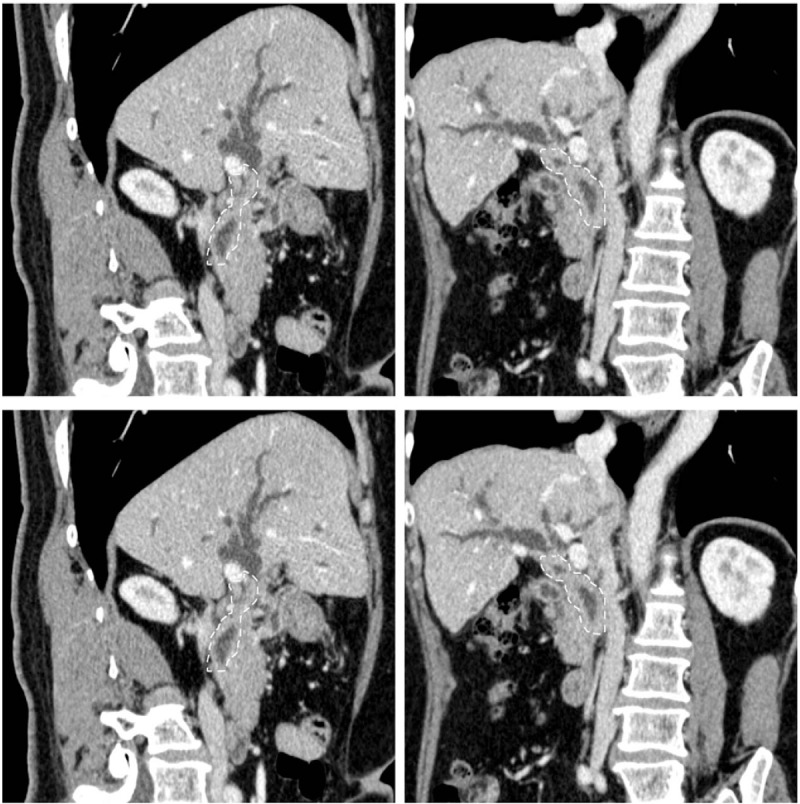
Contrast-enhanced computed tomography with biliary reconstruction showed wall thickening of the entire bile duct and segmental stenosis between 2 dilational parts (dashed line).
